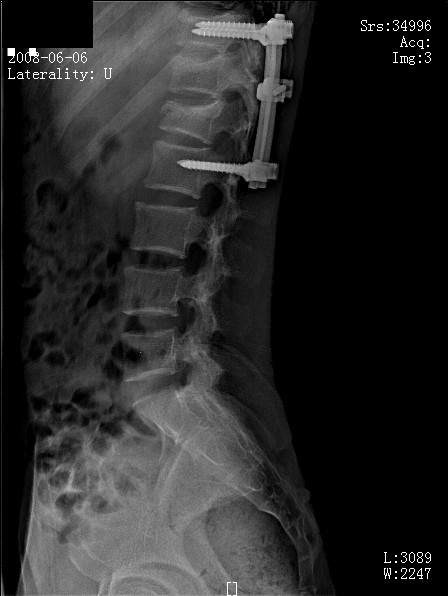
Post-operative radiograph (lateral view) with posterior spinal fusion performed.
